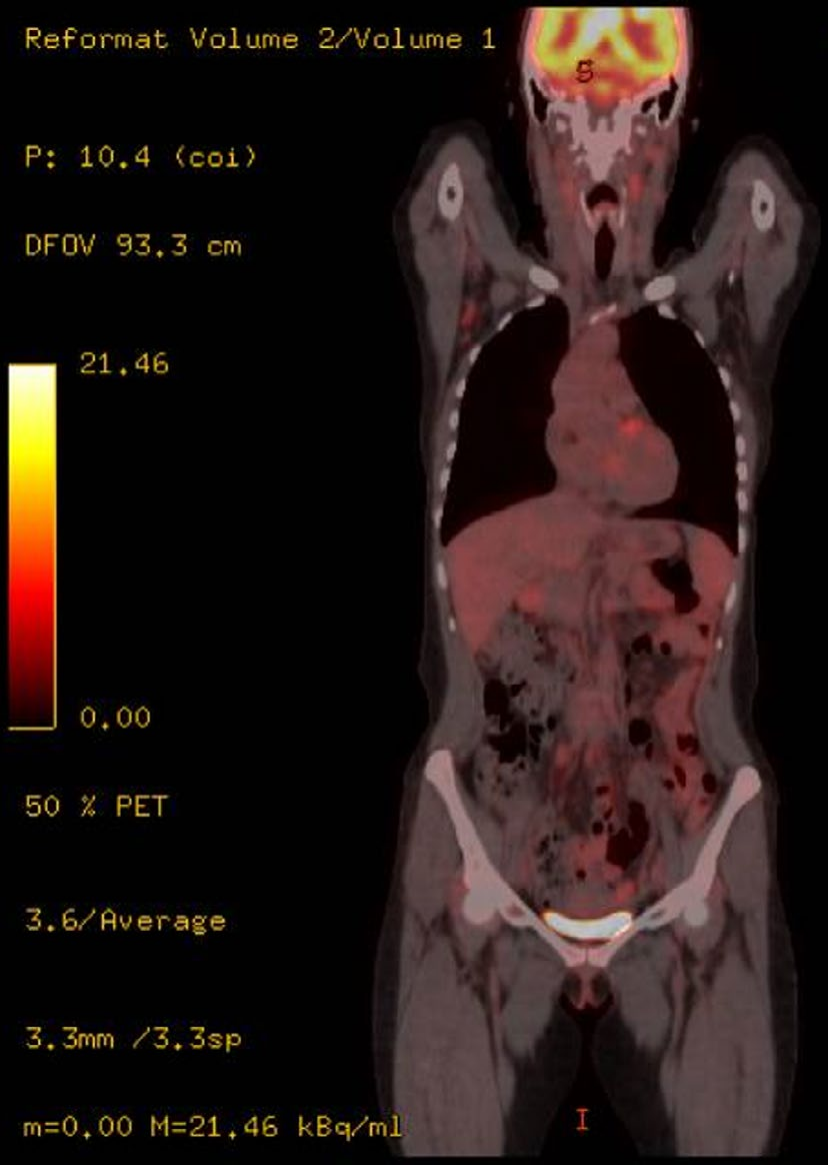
CT/PET scan before BV treatment
